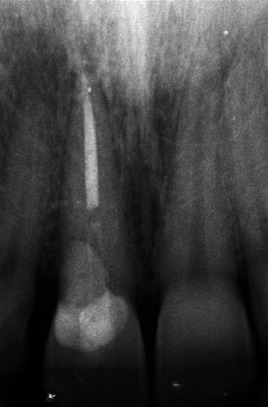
Postoperative X-ray of root canal treatment.
